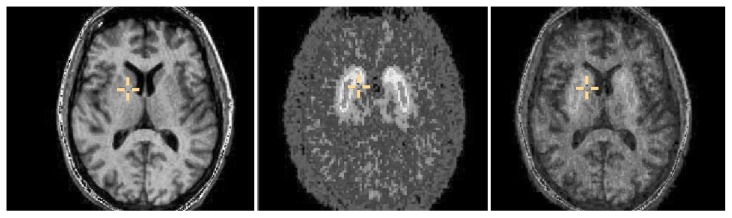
Pictures of the same level of the brain of a 40-year-old male alcoholic. Left: image obtained by magnetic resonance imaging (MRI). Center: view obtained by positron emission tomography (PET) after the administration of the agent [11C]raclopride, which binds to the dopamine receptor. Right: image resulting from the simultaneous combination of MRI and PET. Each picture shows the front of the brain at the top, the back of the brain at the bottom, the left side of the brain at the left, and the right side at the right of the picture. The cross in the images is located between two brain structures: the putamen, to the left of the cross, and the caudate, to the upper right. The MRI image clearly shows the anatomic structures. The PET image demonstrates that both the putamen and the caudate have high densities of dopamine receptors, as indicated by the yellow. However, the borders of these anatomical structures are blurred on the PET image, making them appear as a single structure. Superimposing the MRI and PET images yields an image that facilitates the identification of the distinct borders of anatomical structures such as the putamen and the caudate.
